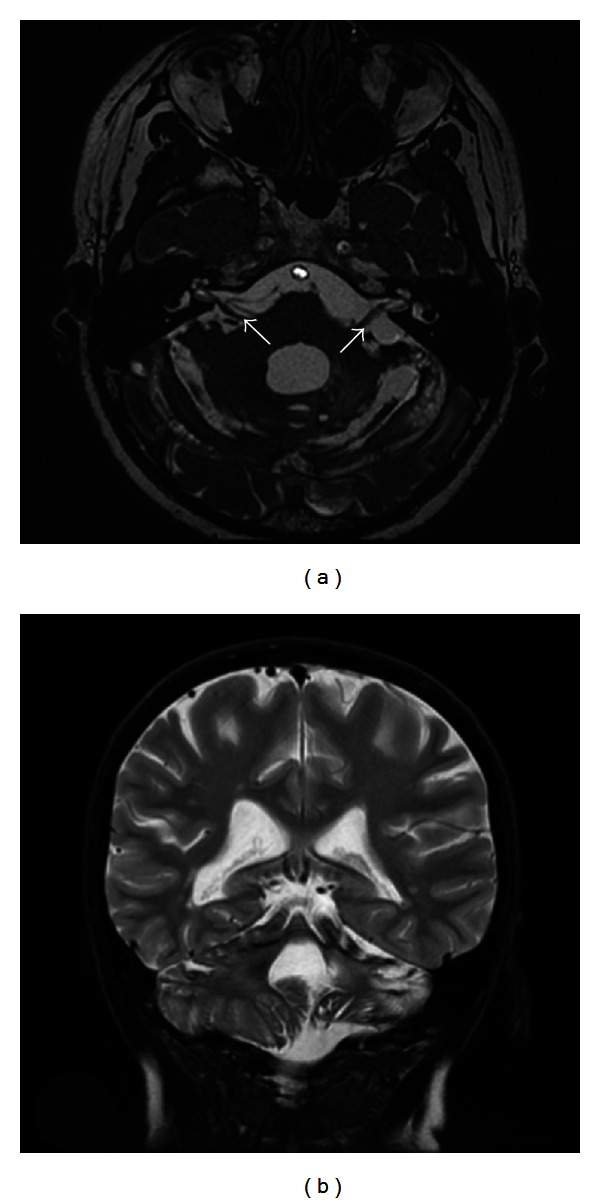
T2-weighted FIESTA magnetic resonance images of superficial siderosis. (a) Axial view of hypointense bands encompassing the vestibular cochlear nerves bilaterally and the cerebellar folia, suggestive of superficial siderosis. (b) Coronal view of cerebella atrophy.
